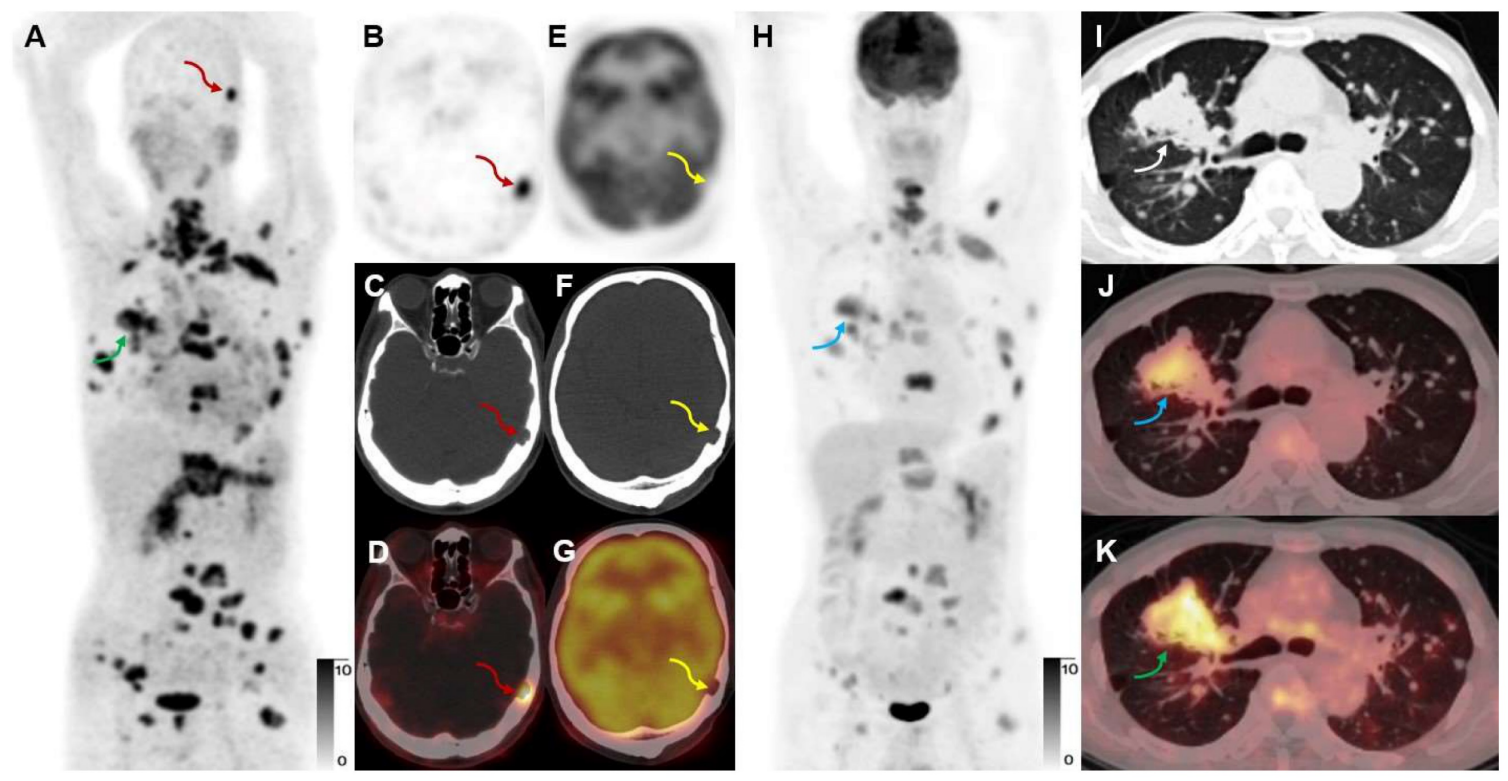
A 65-year-old male participant (Participant No. 9) was diagnosed with IAC with multiple lung, bone, and lymph node metastases at presentation. [^68^Ga]Ga-FAPI-RGD (A, K, green arrows) showed more lesions and a higher T/B ratio than [^18^F]FDG (H, J, blue arrows). Due to the low expression of FAP in the brain, a skull metastasis was clearly visualized on [^68^Ga]Ga-FAPI-RGD PET (A-D, red arrows, SUVmax 15.2), which showed no significant uptake on [^18^F]FDG PET (E-H, yellow arrows; the sections appeared different due to different head positions and the skull metastasis could be seen on CT alone which confirmed that these are comparable slices).
